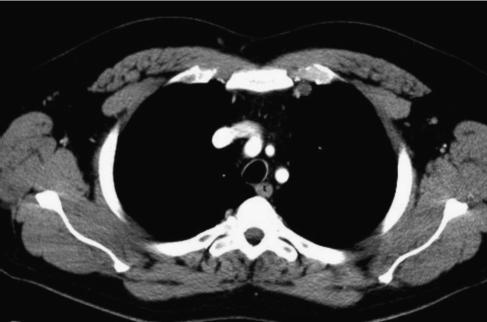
1.2 cm left internal mammary lymph node; unchanged on post chemotherapy chest CT, cause uncertain but it would be an unusual site for seminoma metastases.
